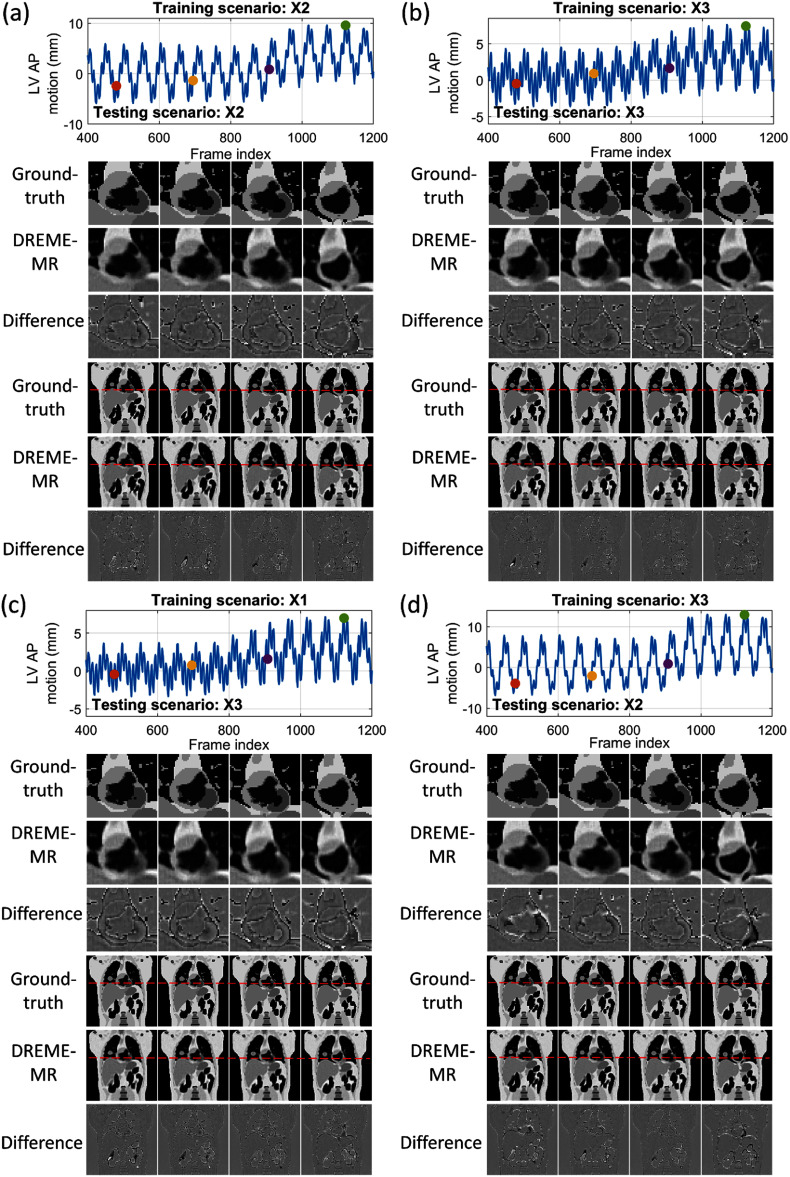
Representative examples of (a), (b) dynamic reconstruction and (c), (d) real-time imaging of the DREME-MR models in the XCAT study. The training and testing scenarios are labeled in each sub-figure. For each sub-figure, the first row shows the estimated LV motion curves along the AP direction, with the dots indicating the selected time points for plotting. The following rows compare the estimated MR images with the ‘ground-truth’ images at the four time points. Rows 2–4 are magnified views of the heart, corresponding to the full-volume images in rows 5–7. The window widths of the difference images are half of those of the MR images.
